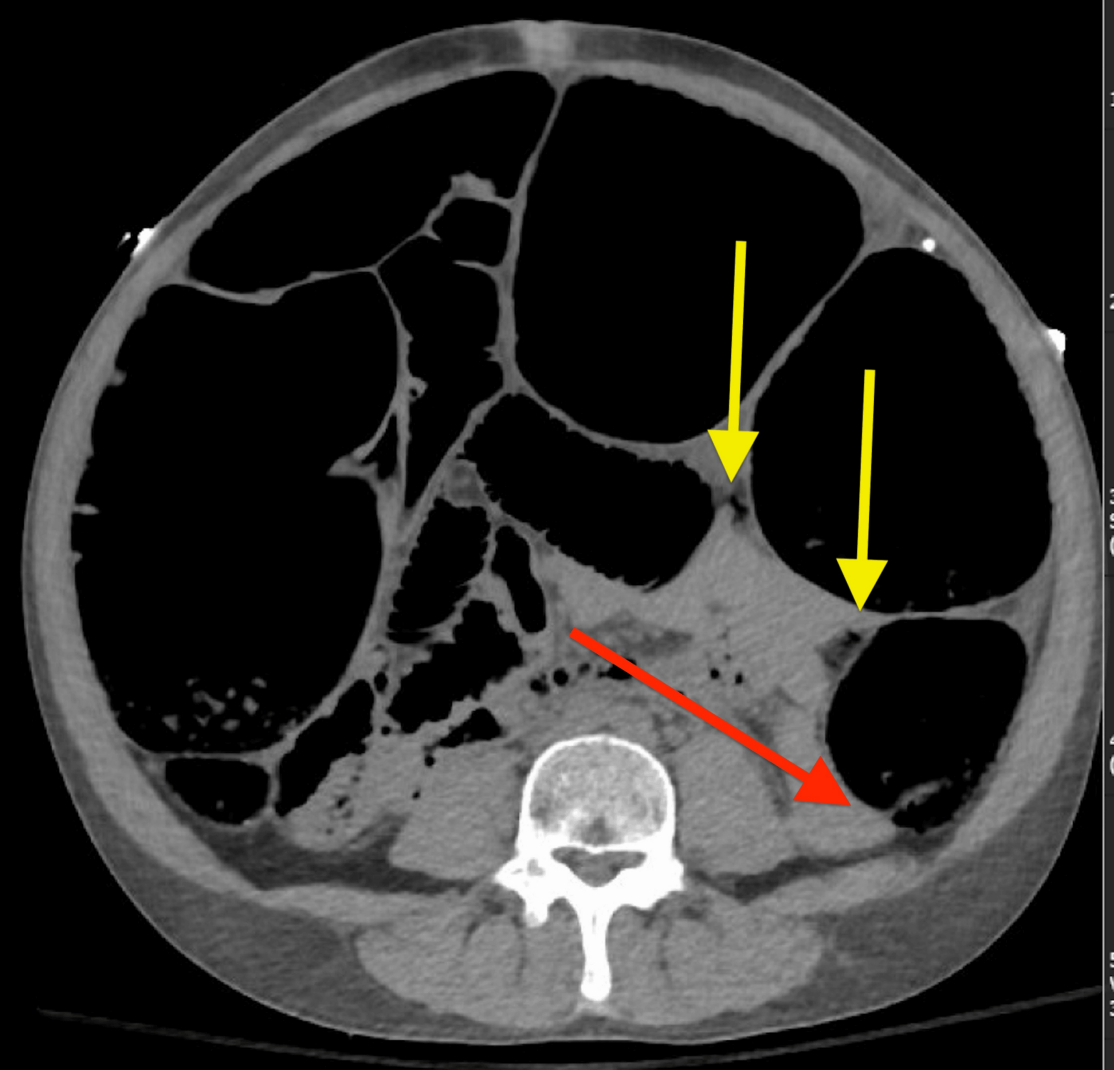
CT abdomen-pelvis on admission, suspicious for pneumatosis coli. Red arrow: Distal tapering of marked colon dilatation near the sigmoid junction, concerning for obstruction, Yellow arrows: multiple foci of air along the descending colon, concerning for visceral perforation.
